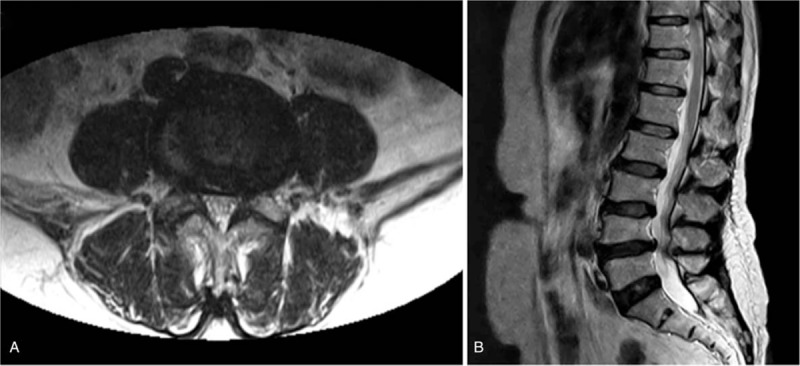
T2-weighted magnetic resonance images of the lumbar spine. (A) Axial image at the lumbar 4-5 level; (B) coronal image of the lumbar spine.
